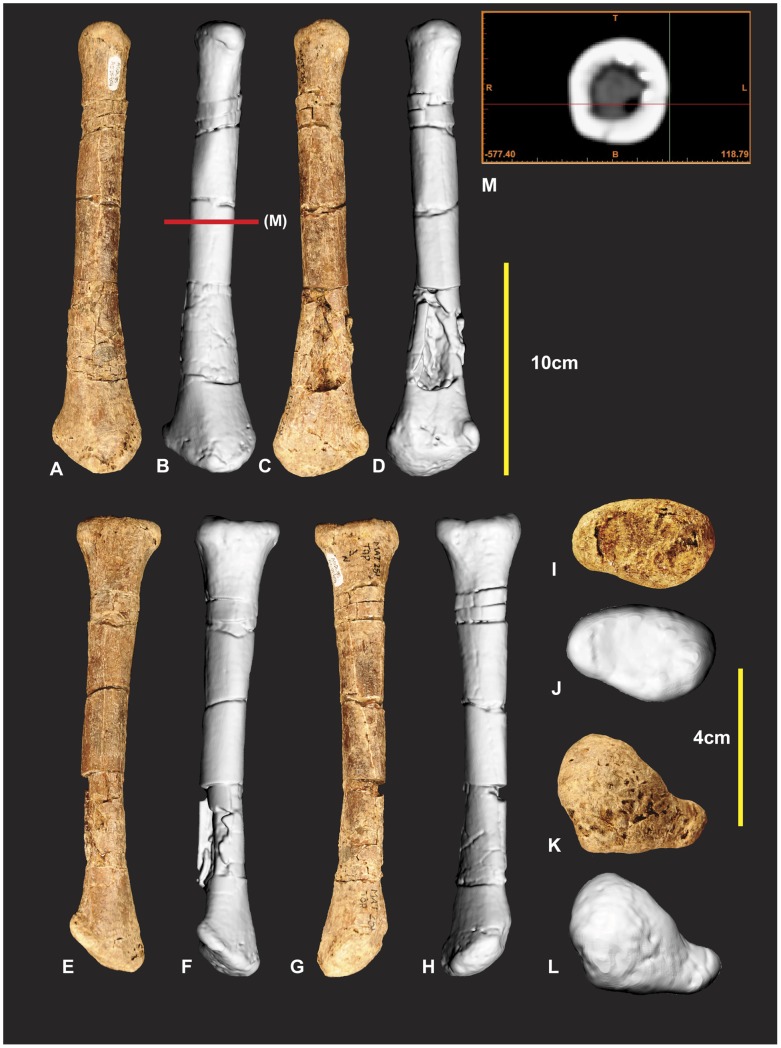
Left Radius. Left Radius in cranial (A & B), caudal (C & D), lateral (E & F), medial (G & H), proximal (I & J), distal (K & L) views, mid shaft CT image (M).
